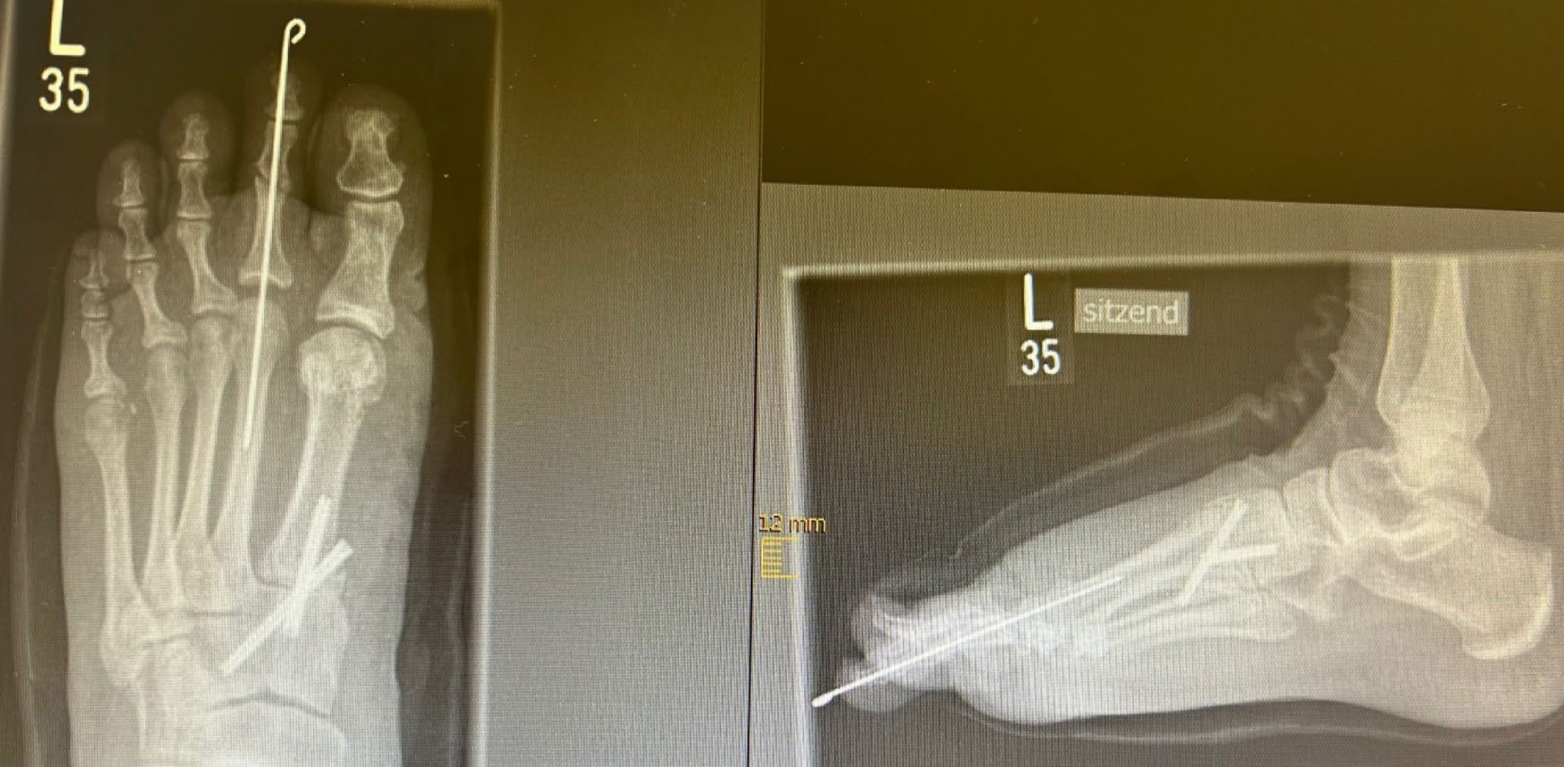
X-ray: postoperative results after open surgical correction of the 2nd ray
